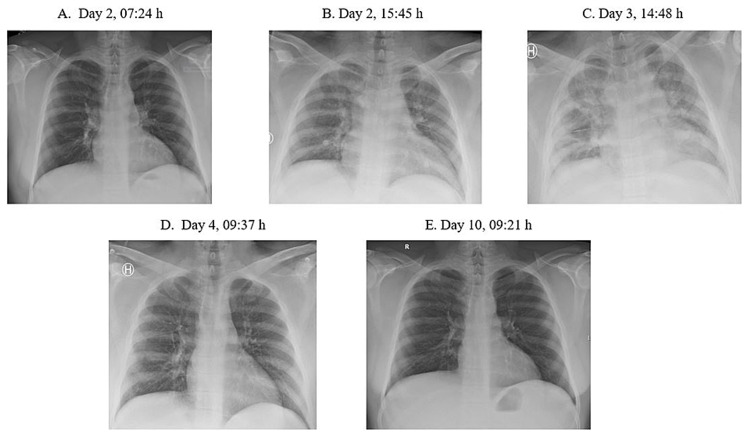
Images of chest x-rays showing the development (A-C) and subsequent regression of pulmonary infiltrates (D, E)
